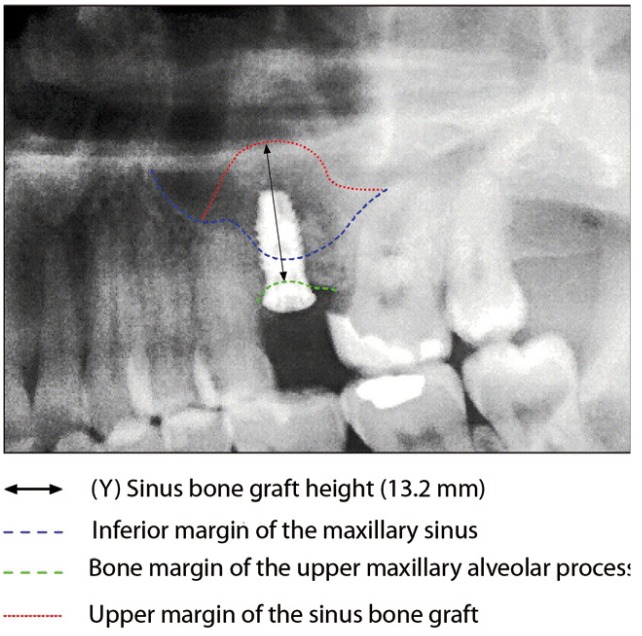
Measurement of bone height gained from the postoperative panoramic X-rays. Total maxillary sinus elevation.
